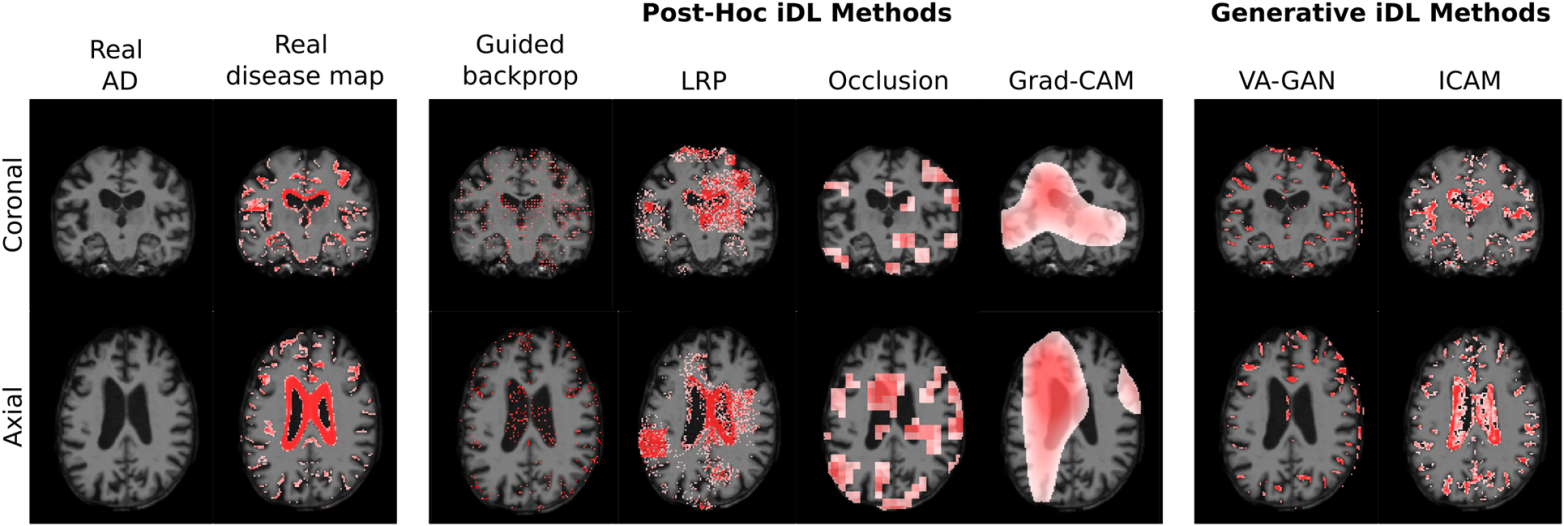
Comparison of post-hoc interpretability maps and generative interpretability methods applied to the classification of Alzheimer’s disease (AD) versus Mild cognitive impairment (MCI) in brain MRI volumes. The real disease map is the “ground-truth” shown for comparison. Figure adapted fromBass et al. (2022).
